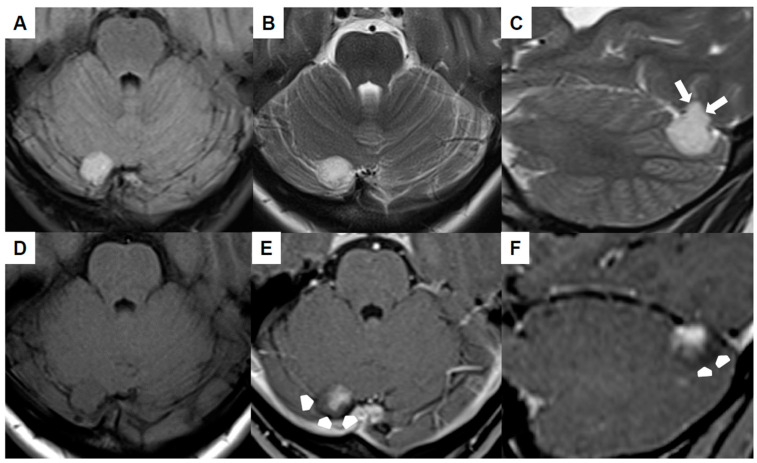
Preoperative magnetic resonance imaging of Case 1: The venous hemangioma showed a hyperintense signal in the fluid-attenuated inversion recovery (A) and T2-weighted sequences (B,C) with signs of tentorial infiltration (arrows in (C)). The lesion was cortex-isointense in the unenhanced T1-weighted sequence (D) and yielded a relatively homogenous contrast enhancement except for the dorsal margins (arrow-heads in (E,F)).
